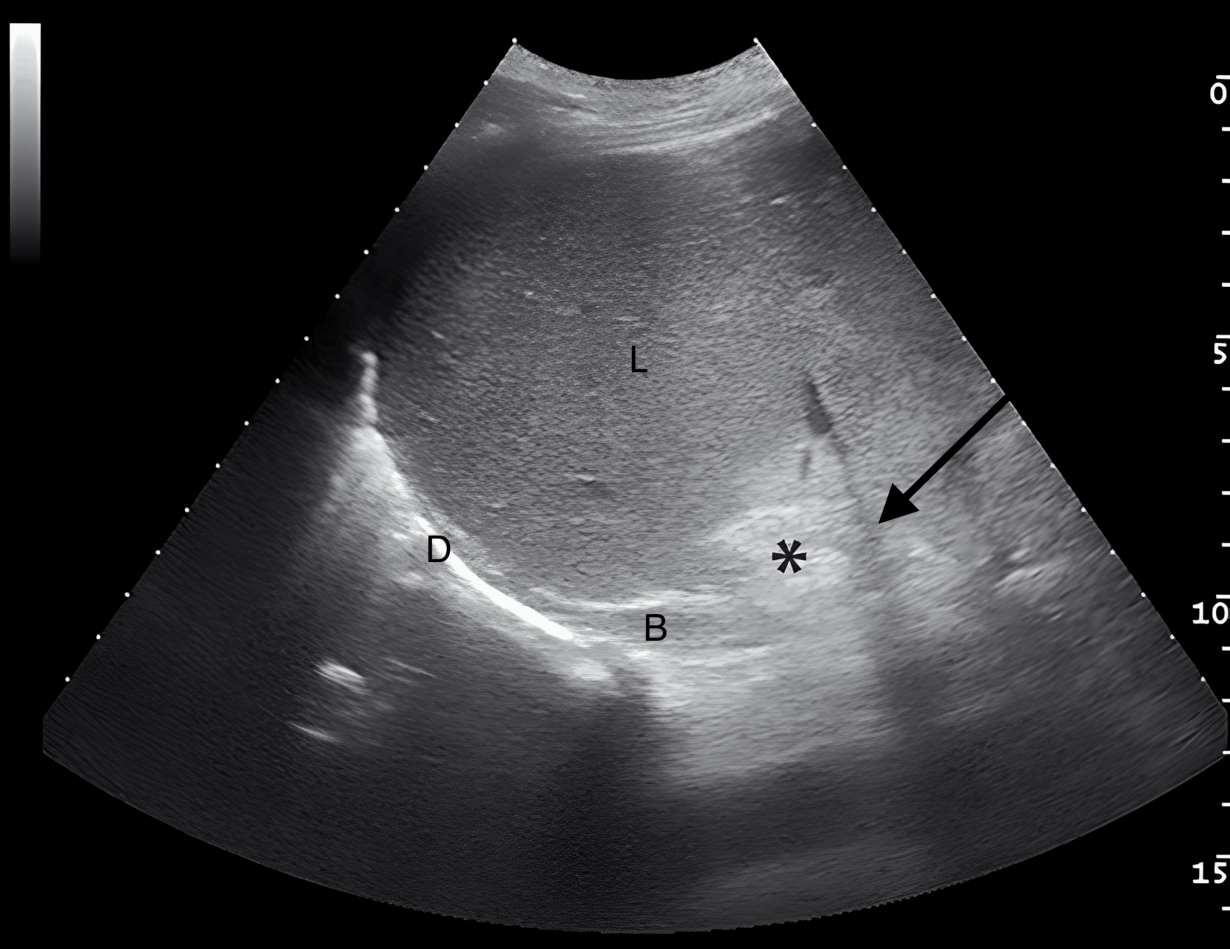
Ultrasound image of the right upper quadrant demonstrating the liver (L), inferior vena cava (arrow), and a pericaval haematoma (*). Perihepatic blood (B) is visible below the diaphragm (D)
